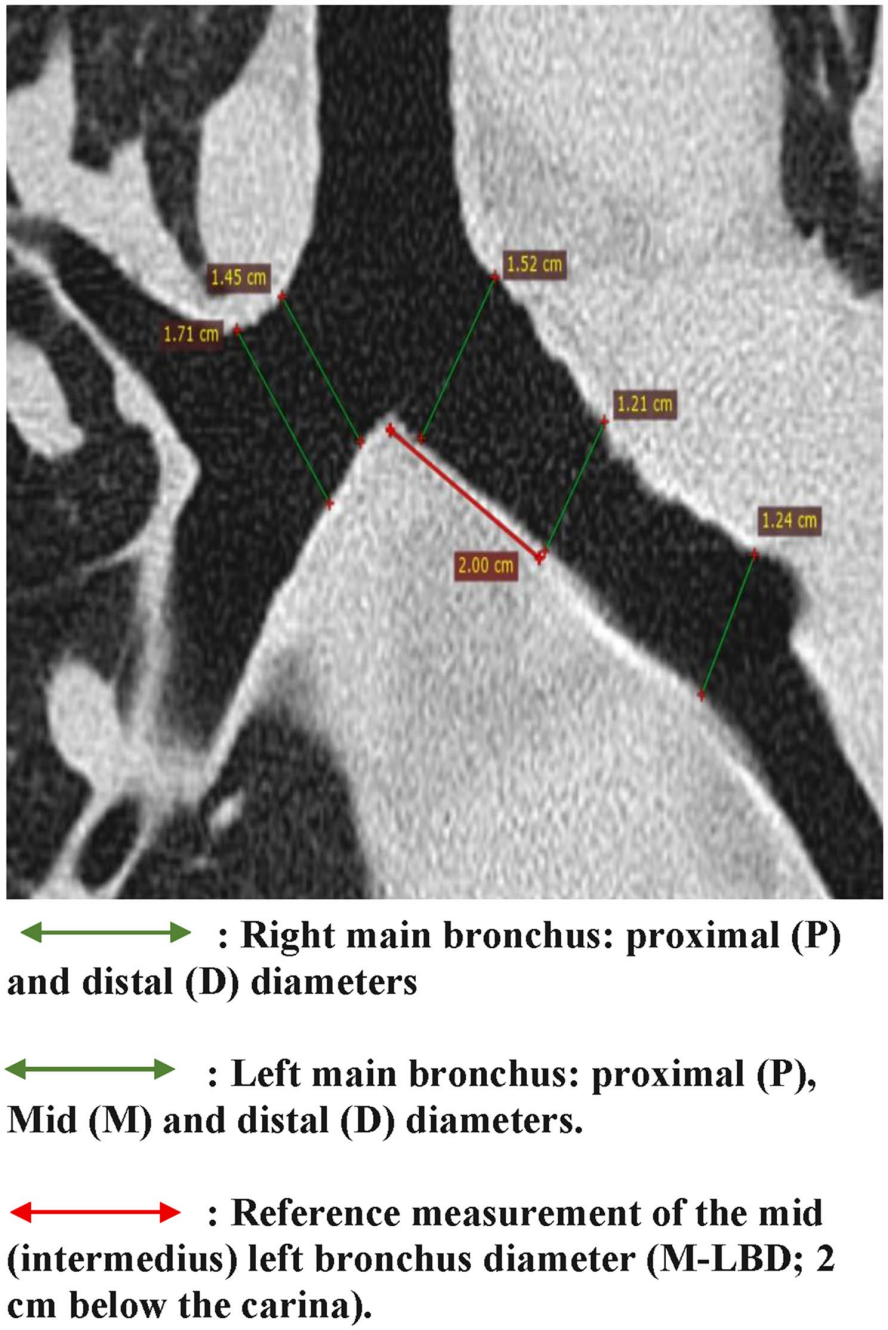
Coronal chest CT reconstruction (lung window) demonstrating main bronchus diameter measurements
